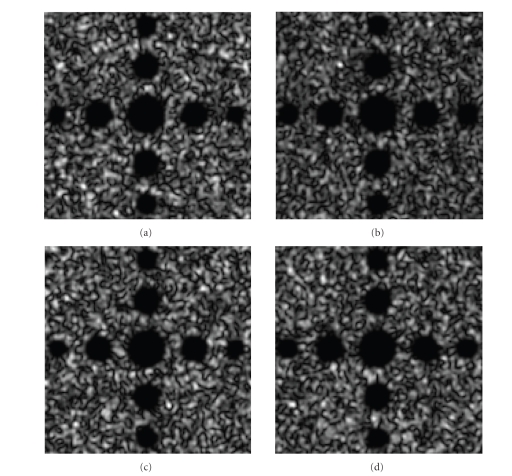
Two synthetic 3D ultrasound image sets
